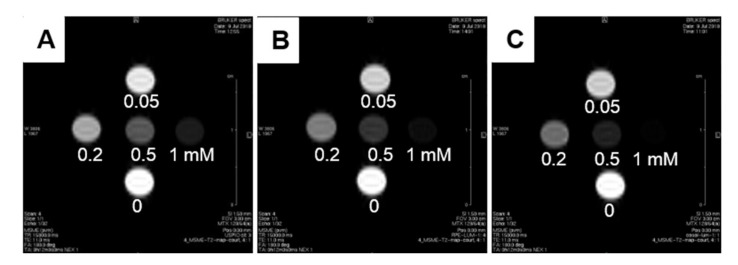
T2* weighted MRI at 7 T of: (A) free MNPs; (B) REV_MCLs; and (C) cosol_MCLs at various iron concentrations in water.
